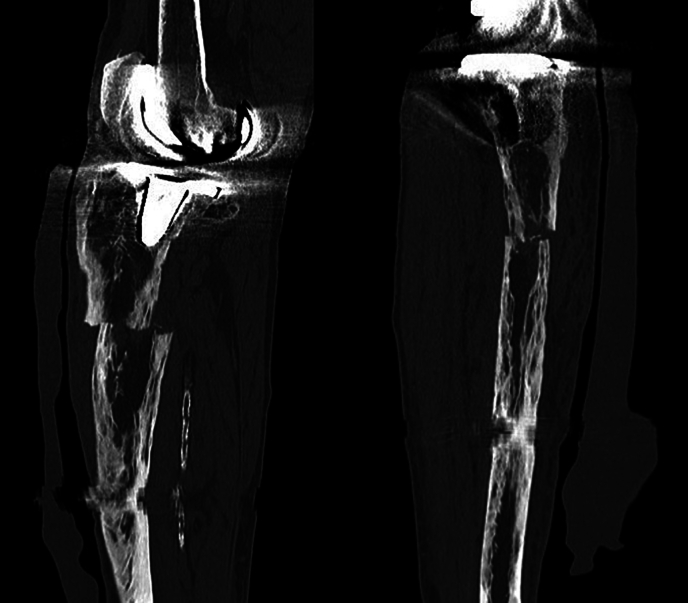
Sagittal and coronal CT scan planes showing diffuse osteosclerotic and osteolytic changes in the proximal tibia and tibial shaft.
